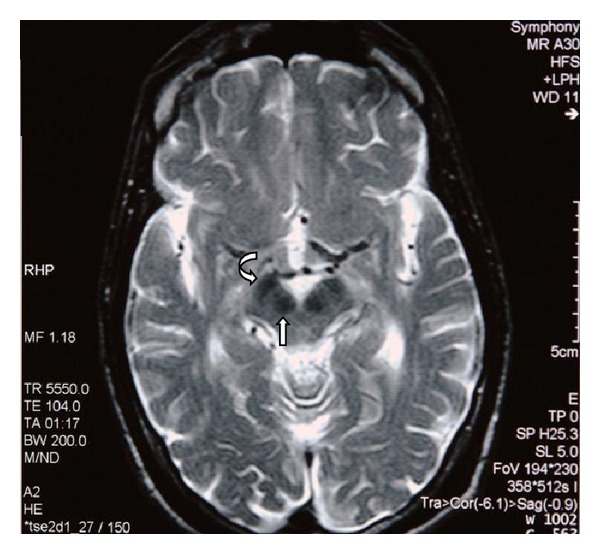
Axial T2 weighted MR image of the brain at the level of the midbrain reveals hypointensity of the red nuclei (straight arrow) and substantia nigra (curved arrow).
